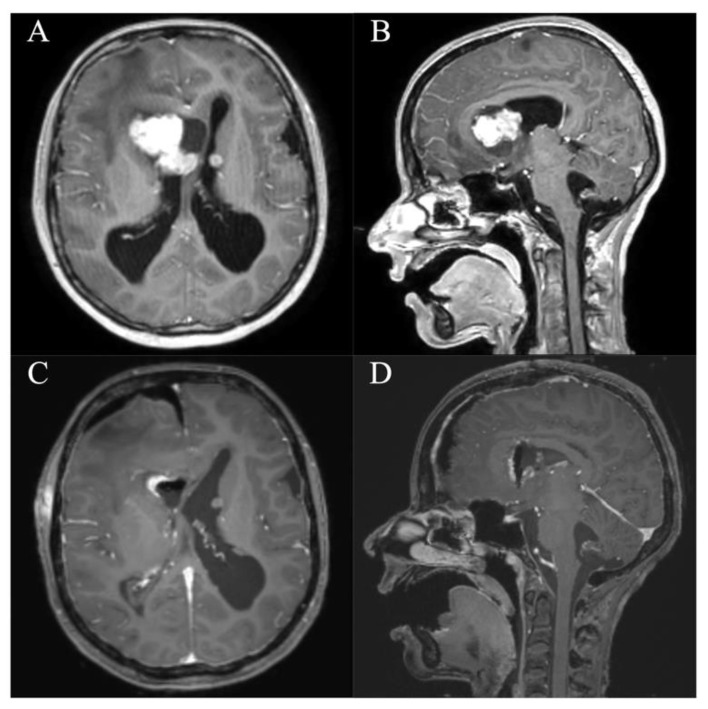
Case 7. Axial and sagittal MR images, T1-weighted sequences with contrast medium preoperatively (A,B) and immediately postoperatively (C,D) after resection of the intraventricular subependymal giant cell astrocytoma via a right frontal endoscopic-assisted approach. It shows a gross total resection with residual blood in the resection cavity. Furthermore, there are pre-existing subependymal tuberosities in both lateral ventricles.
